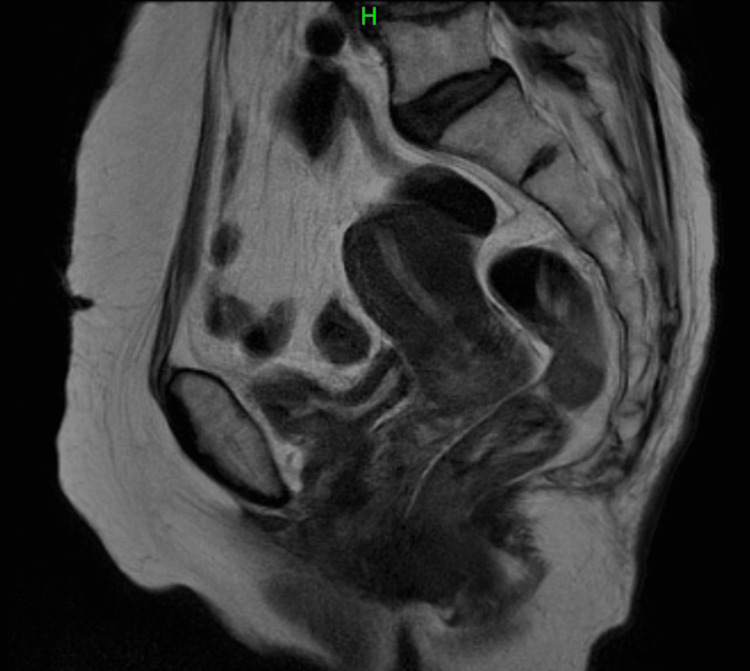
Pelvic MRI examination Pelvic MRI showed no endometrial thickening, and imaging studies were negative for uterine lesions, including endometrial cancer. Imaging studies were also negative for ovarian tumors.
